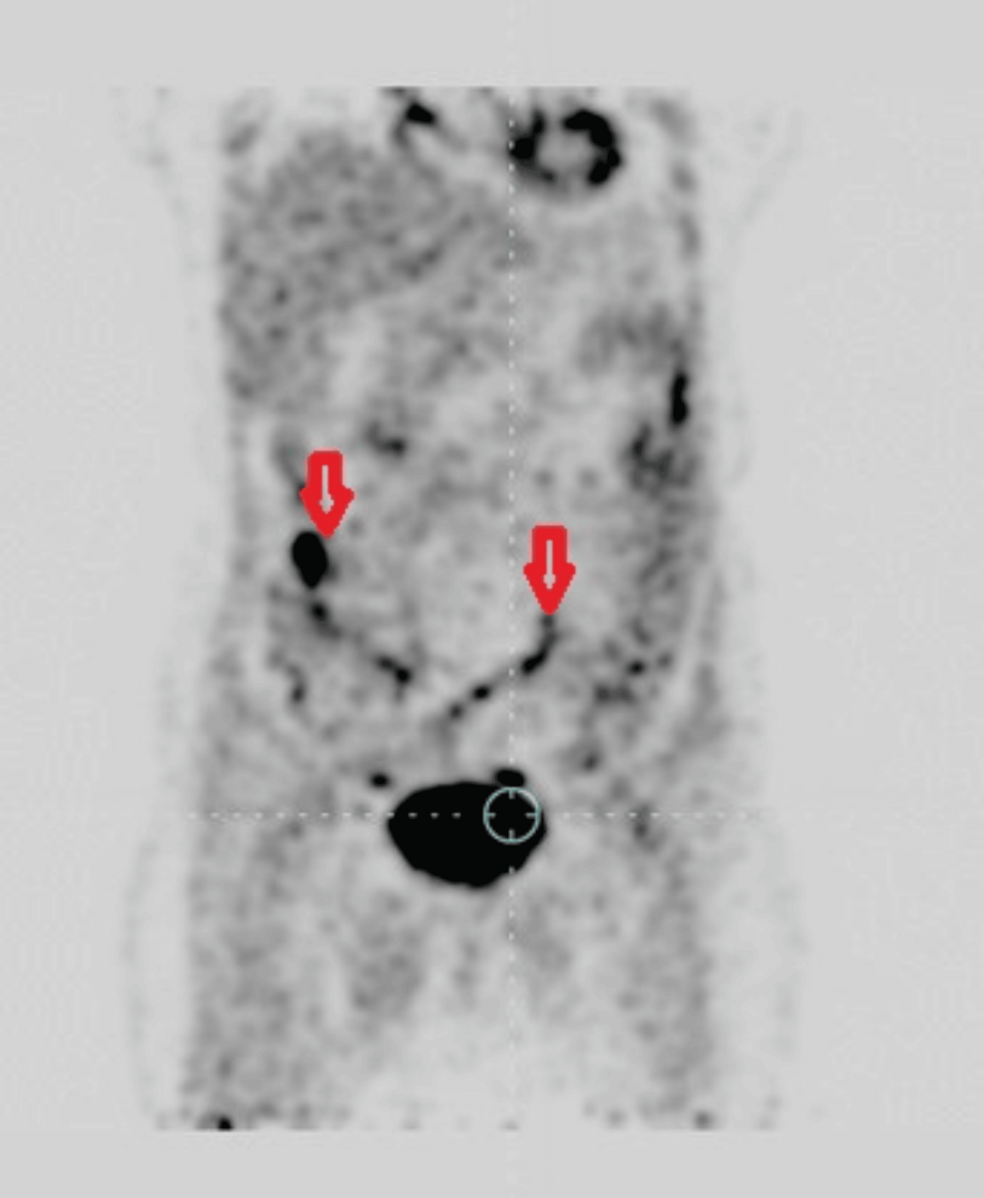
CECT photograph showing enlarged pelvic lymph nodes (Case 4). CECT: contrast-enhanced computed tomography.
